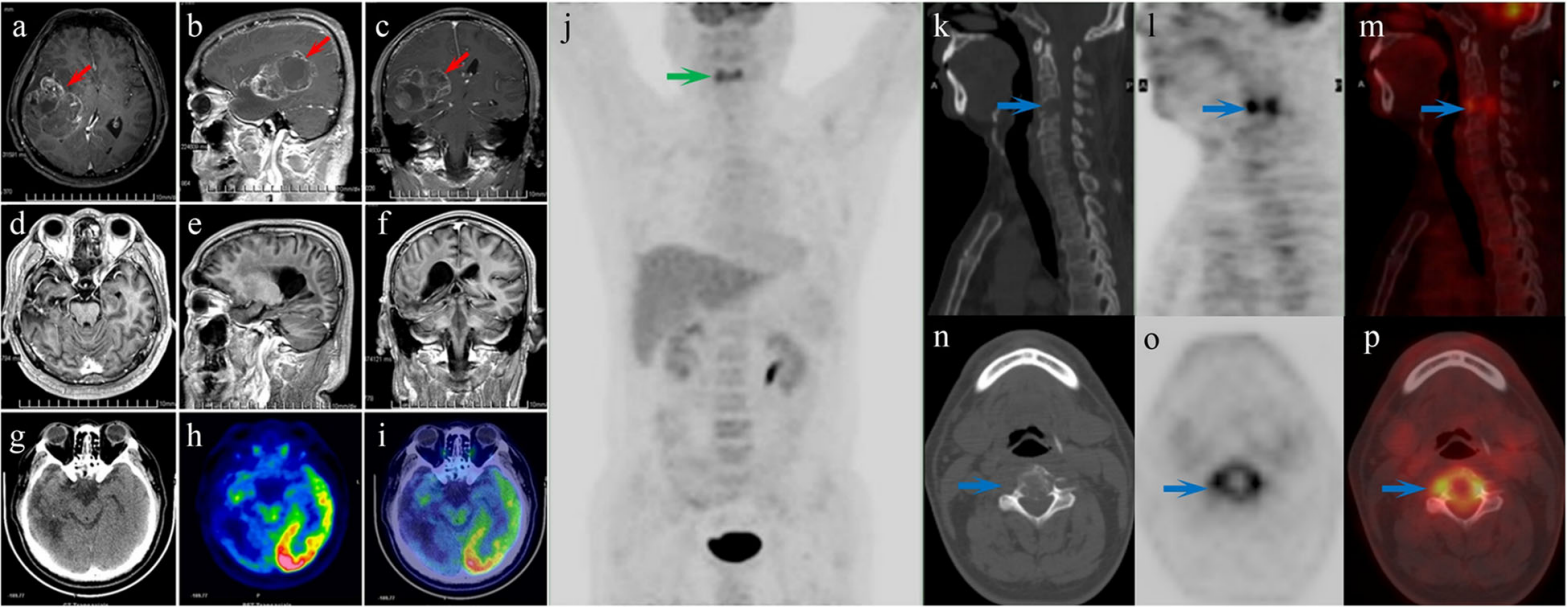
a-c Presurgical T1-weighted MR images showed a large, lobulated, and ill-defined 7.8 cm × 5.3 cm × 4.0 cm mass with inhomogeneous enhancement in the right temporal lobe (red arrows). The involvement of right lateral ventricular was also observed. d-f Follow-up postcontrast MR and (g-i) brain FDG PET /CT images indicated the postoperative changes without any signs of local recurrence at the resection cavity. (j) Whole-body FDG PET/CT anteroposterior 3-dimensional maximum intensity projection (3D-MIP) image showed a focal abnormal FDG-avid lesion in the cervical region without any other positive findings in the body (green arrow). (k-p) The selected sagittal and transaxial views of PET/CT images demonstrated a solitary intense FDG-avid osteolytic lesion associate with a compression fracture in the 4th cervical vertebral body (blue arrows). (o-p) Transaxial view of PET/CT images showed (blue arrow) increased FDG-uptake in the periphery of the mass with a photopenic center, the so-called “doughnut” sign
